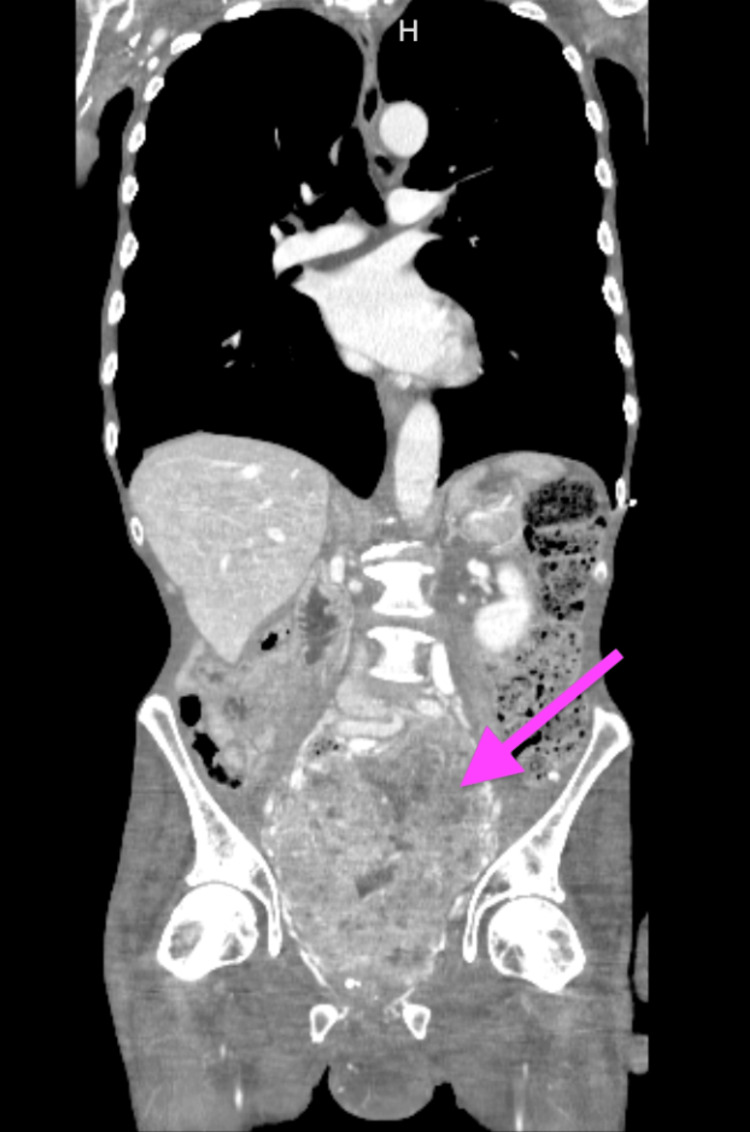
CT chest/abdomen/pelvis demonstrates 15 cm x 12 cm x 15 cm heterogeneously enhancing pelvic mass
